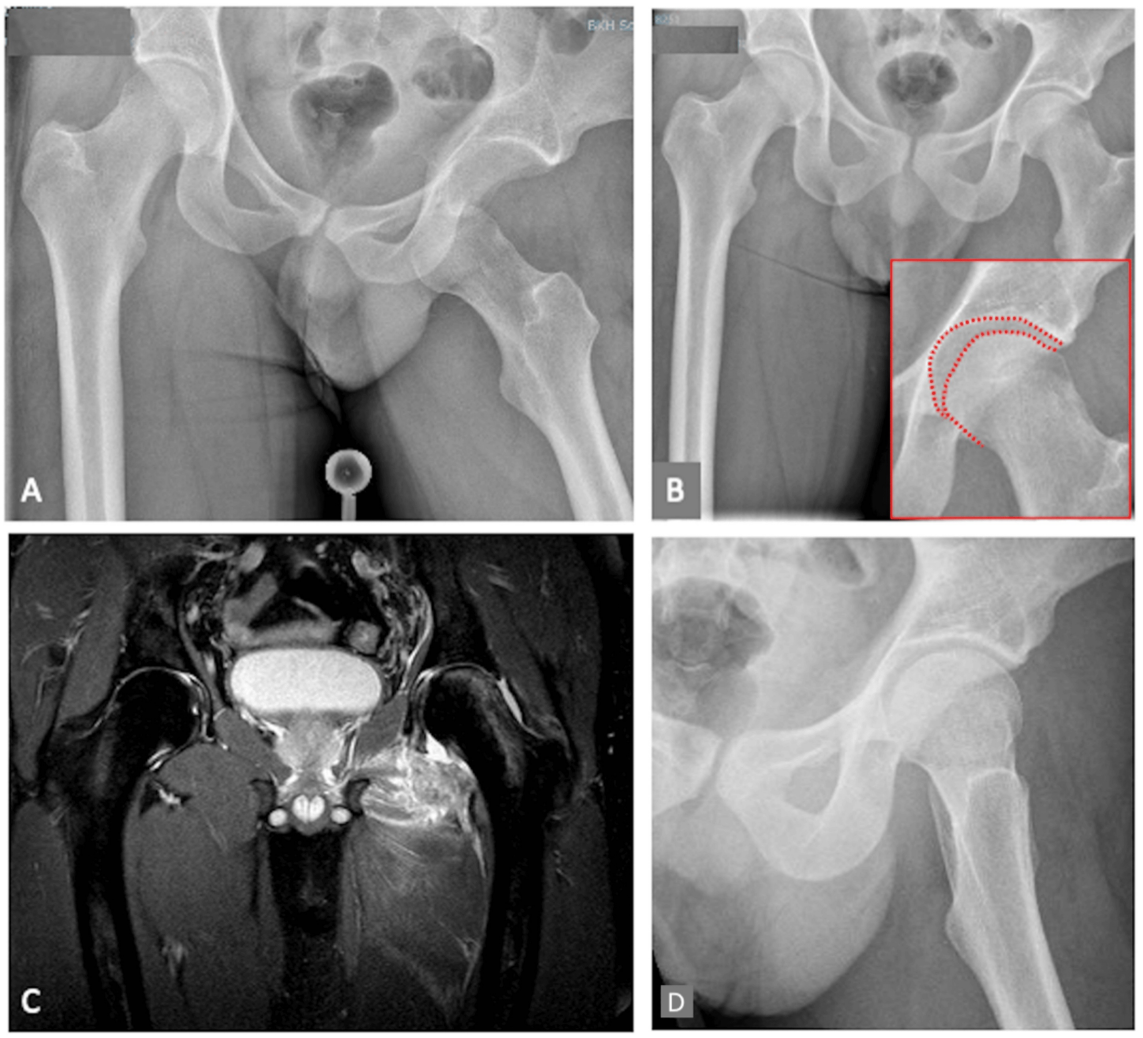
Radiographs of a traumatic obturator hip dislocation (A) and after closed reduction (B) demonstrating an incongruent joint line (red dotted line). The MRI revealed a caudal labral tear with free articular bodies (C). Therefore, arthroscopic labral refixation and removal of the free articular bodies was performed. The postoperative radiograph shows a congruent hip joint (D).
